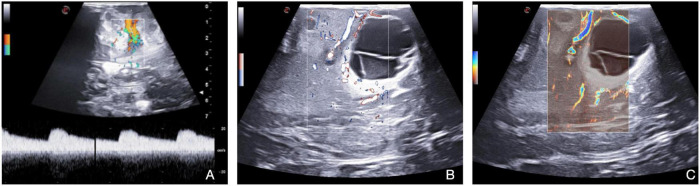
Doppler techniques (Color-Doppler, X-Flow, Micro-V). Color Doppler and spectral Doppler (A) bring information about flow direction and velocity with quantification of the velocity through spectral Doppler. X-Flow (B) maximizes spatial resolution and Doppler signal penetration capabilities. Micro-V (C) permits real-time micro-vascular perfusion analysis, identifying low flow signals.
